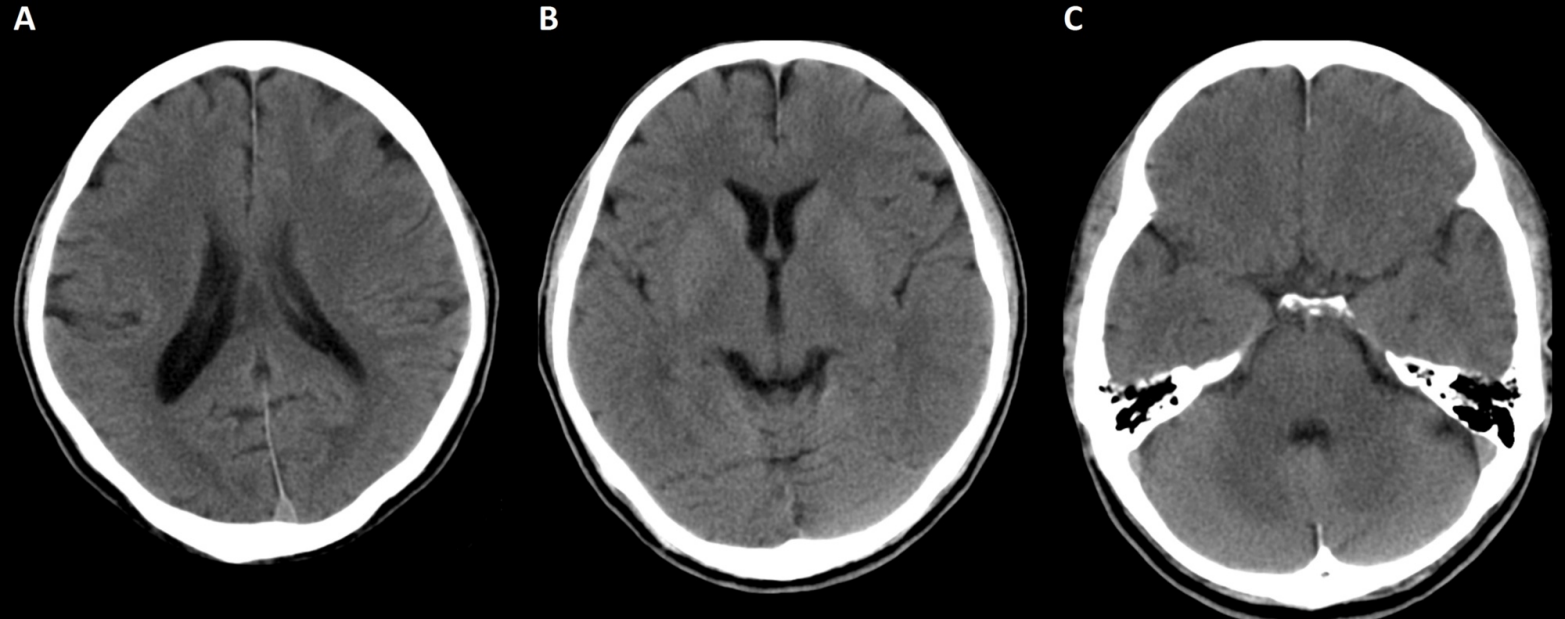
CT of the brain on admission. There is no evidence of acute insult or cerebral edema on the CT after the administration of the Lilly Cyanide Antidote Kit. (A) CT of the brain at the level of the body of the lateral ventricles: frontal, parietal, and occipital lobes are not affected. (B) CT of the brain at the level of basal ganglia: normal caudate nuclei, globi pallidi, and putamina. (C) CT of the brain at the level of cerebellar hemispheres: no evidence of ischemic insult at the temporal and cerebellar hemispheres.
